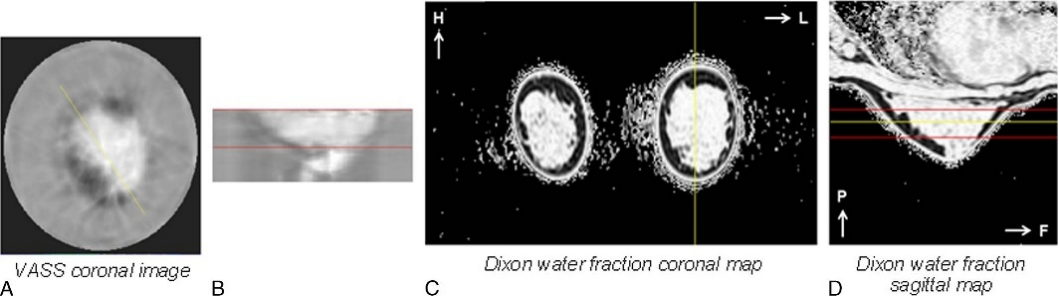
Representative UST images (A and B) and water fraction maps (C and D) of a woman with high breast density (percent water content 68.1%) - with A: Speed of Sound coronal image, B: Speed of Sound sagittal image, C: Dixon water fraction coronal map and D: Dixon water fraction sagittal map. The yellow lines indicate the relative position of depicted coronal and sagittal slices; the red lines indicate the matched coronal limits of UST and MRI VOI and the corresponding volumes where VASS and water content were calculated.
